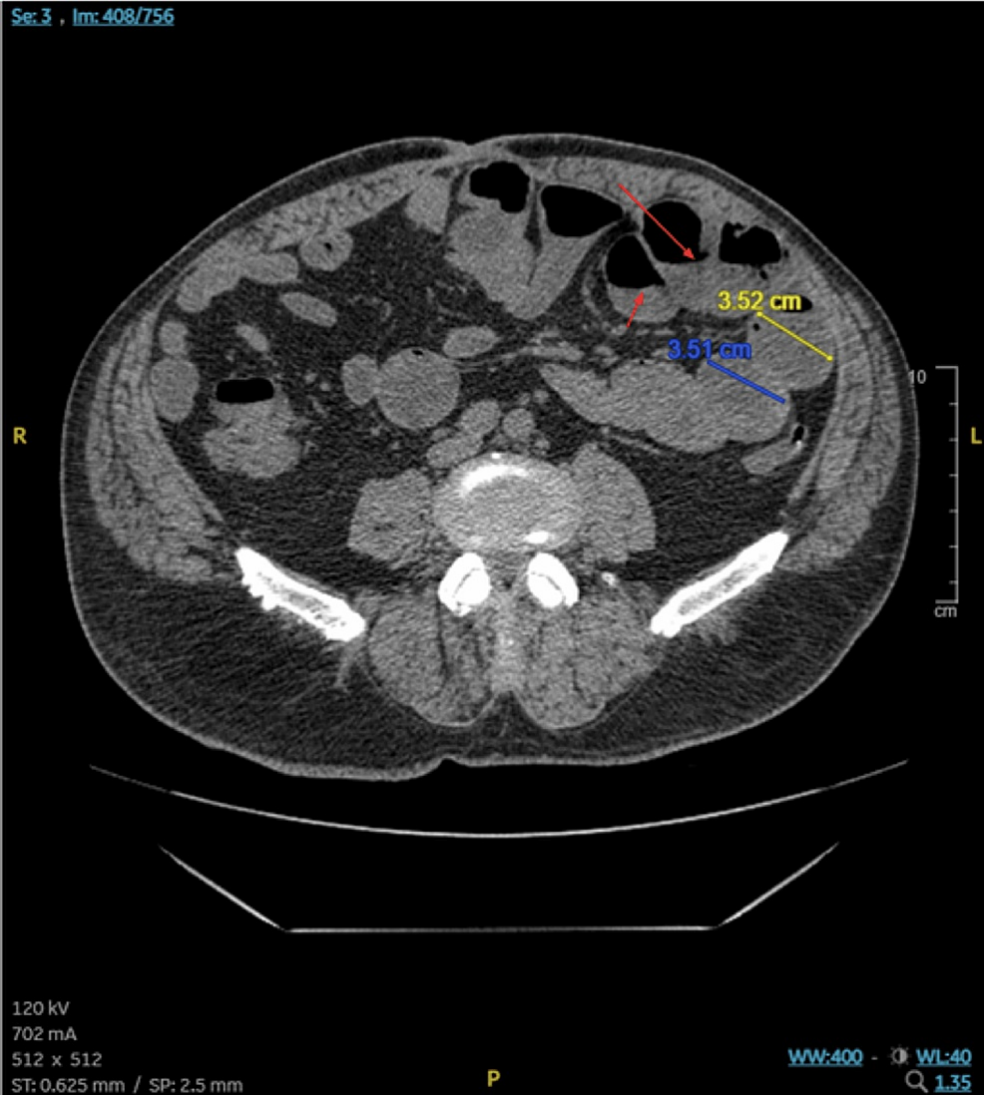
CT scan of the abdomen Remarkable for small bowel obstruction with dilated loops of small bowel measuring up to 3.5cm (examples indicated by yellow and blue lines with respective measurements). Fluid levels are seen within the colon (red arrows).
